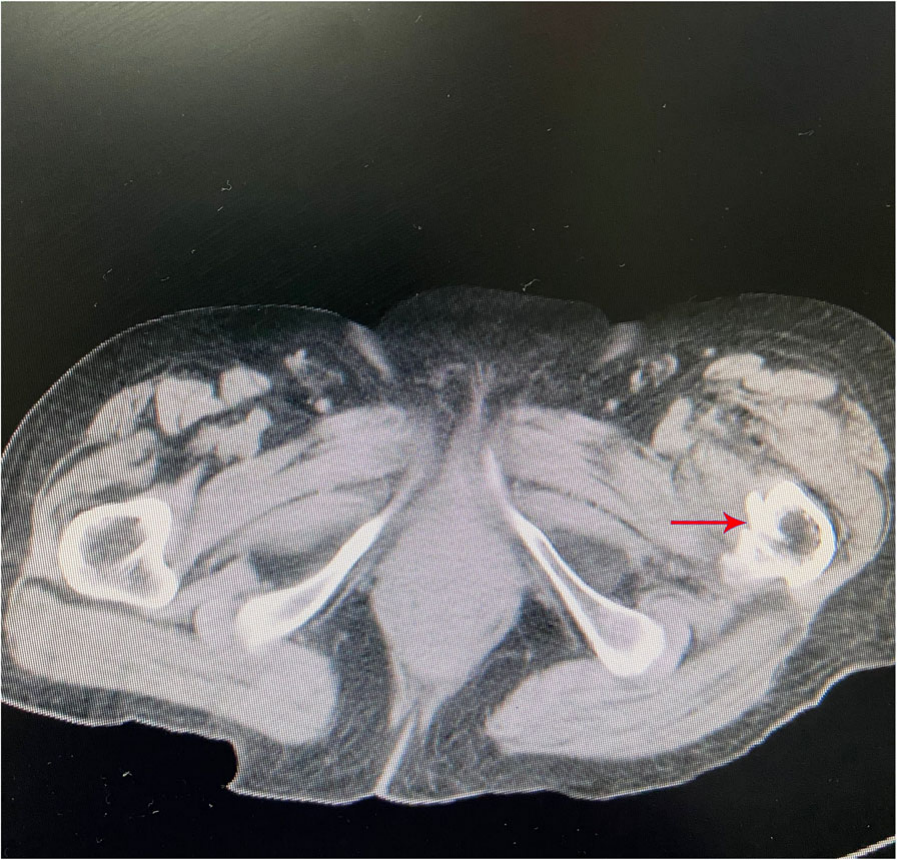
Destruction of the calcar femorale (red arrow) in cross-sectional computed tomography image of the right hip with an intertrochanteric fracture
